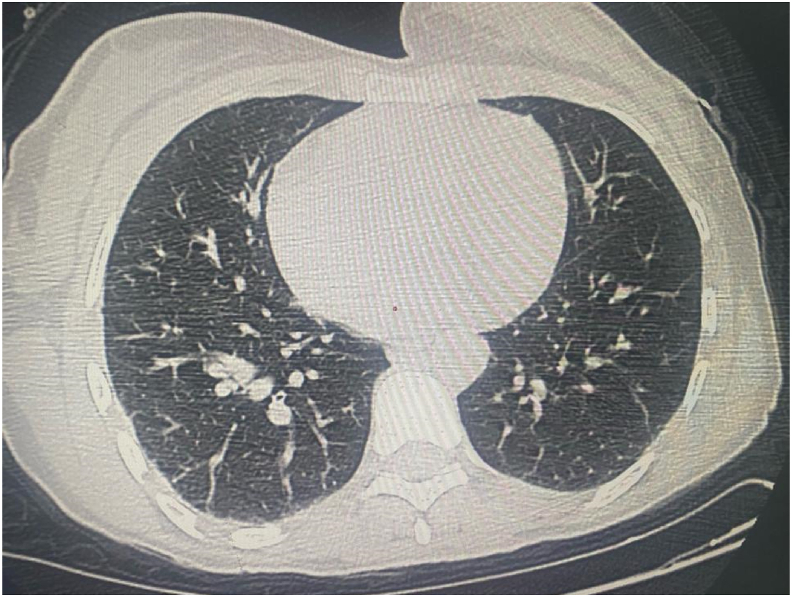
CT image not showing a SARS-CoV-2 type attack.
